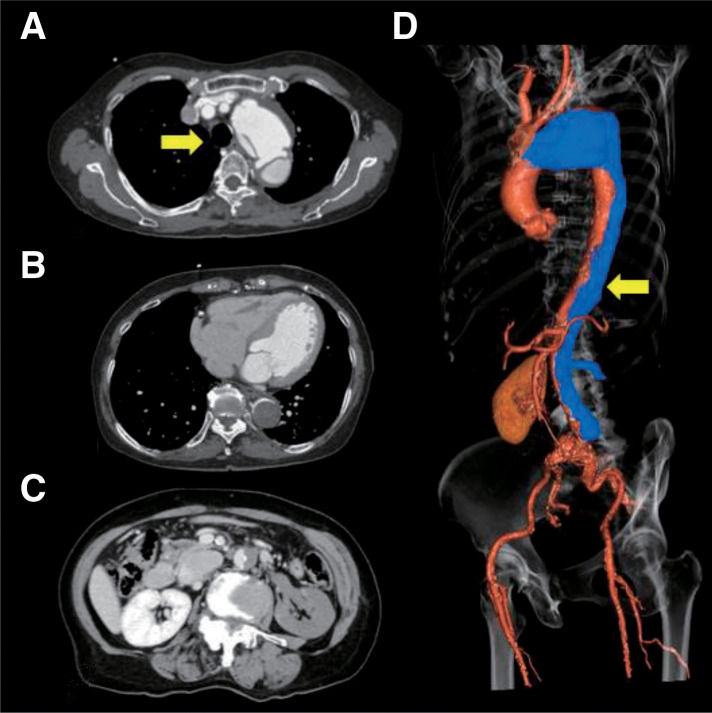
Fig. 1 Preoperative CT scan. Preoperative contrast-enhanced CT demonstrated an acute type B aortic dissection with a patent false lumen within the distal aortic aneurysm (A, arrow). The false lumen within the aneurysm remained patent. At the same time, the mid-descending thoracic aorta and distal segments exhibited a thrombosed false lumen type, and the true lumen below the descending aorta was compressed (B). The left renal artery was not enhanced in the late phase (C). The arrow indicates the false lumen in the 3D reconstructed image (D). 3D: 3 dimensional; CT: computed tomography
